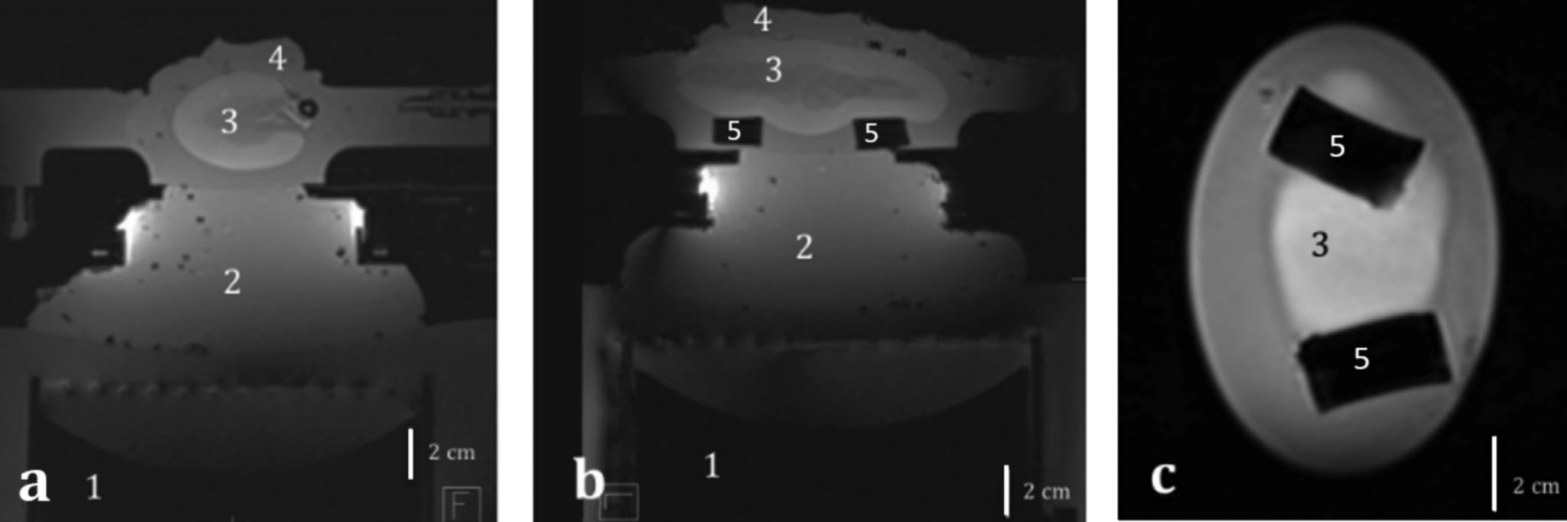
T1 MR images of an excised ex vivo perfused kidney in (a) transversal, (b) sagittal and (c) coronal plane. 1. HIFU transducer, 2. Standard ultrasonic gel for acoustic coupling, 3. Perfused organ, 4. Standard ultrasonic gel for acoustic wave dissipation, 5. Soft supports for the organ.
